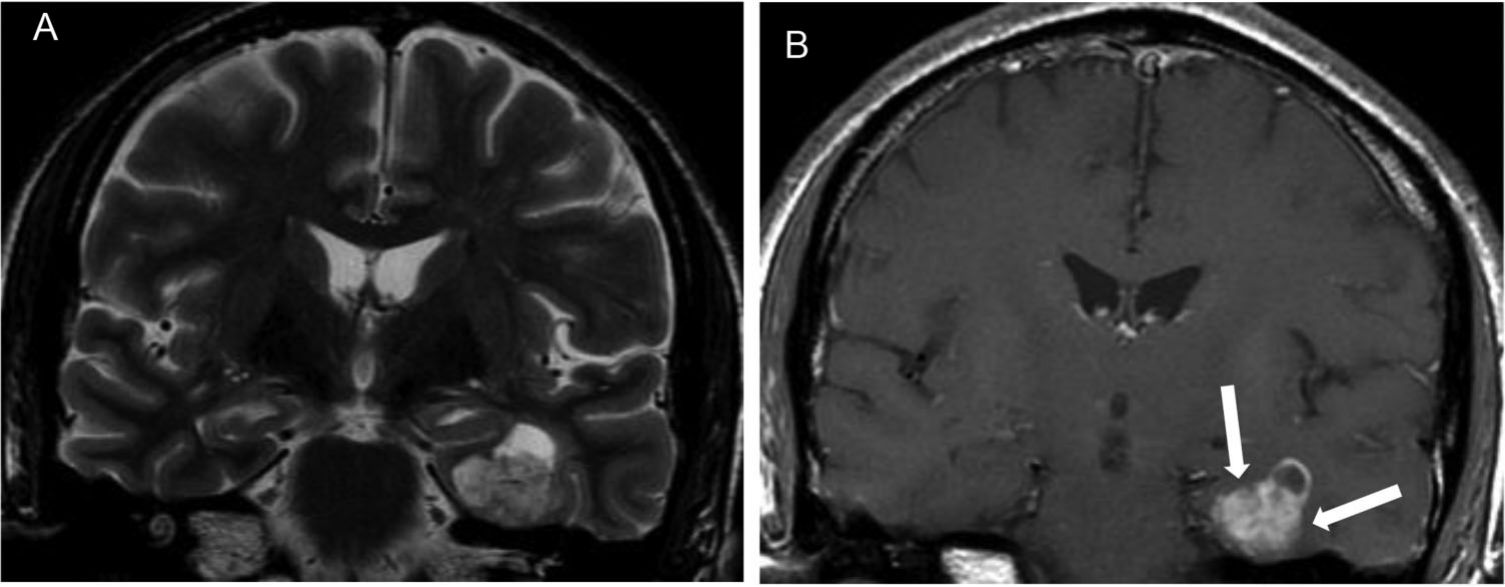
A MRI of a ganglioglioma in a 45-year-old man with refractory left partial mesial temporal lobe epilepsy. A) A coronal T2-weighted image and B) a contrast-enhanced coronal T1-weighted image showing a solid-cystic lesion in the mesial left temporal lobe with intense enhancement of the soft tissue component (white arrows).
